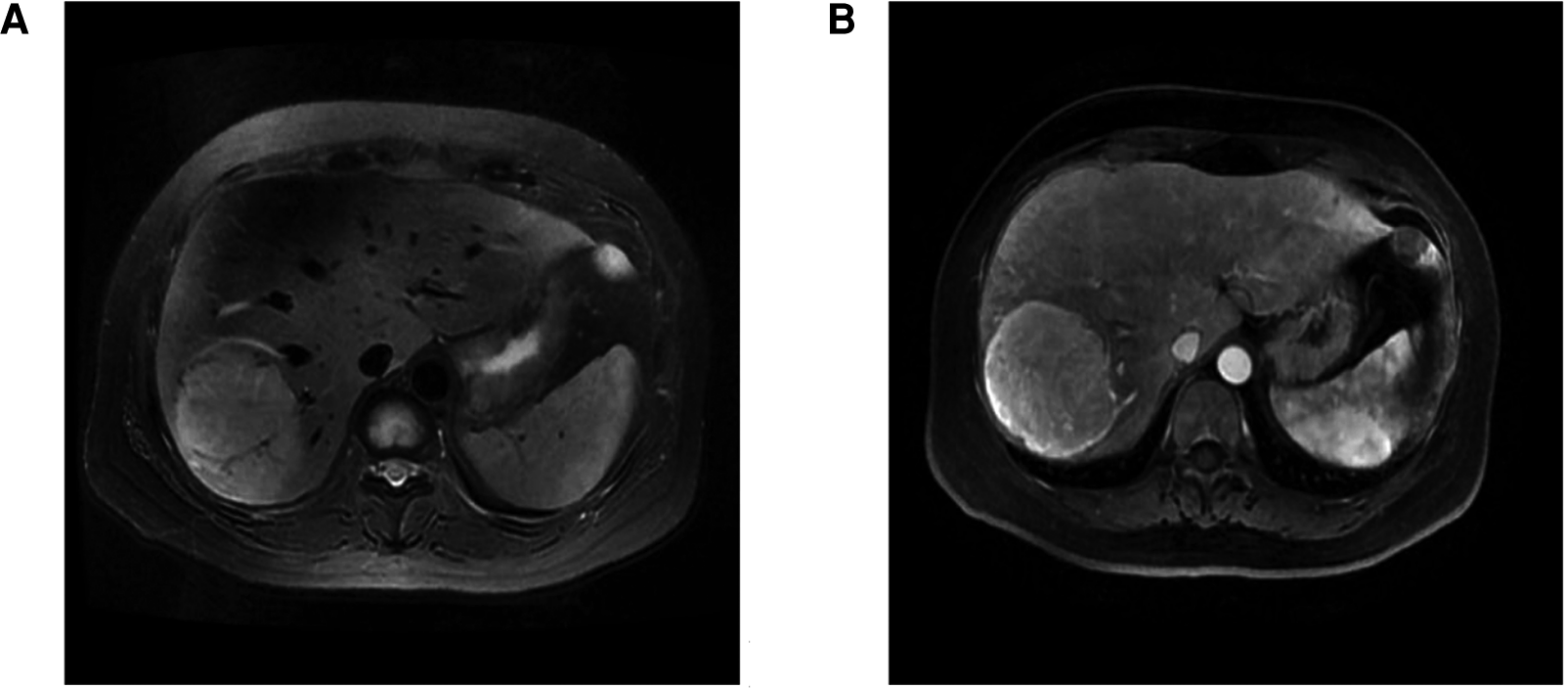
MRI of inflammatory myofibroblastoma of the liver. (A) shows the MRI plain T2-weighted image (T2WI). (B) shows the enhanced scan image.
